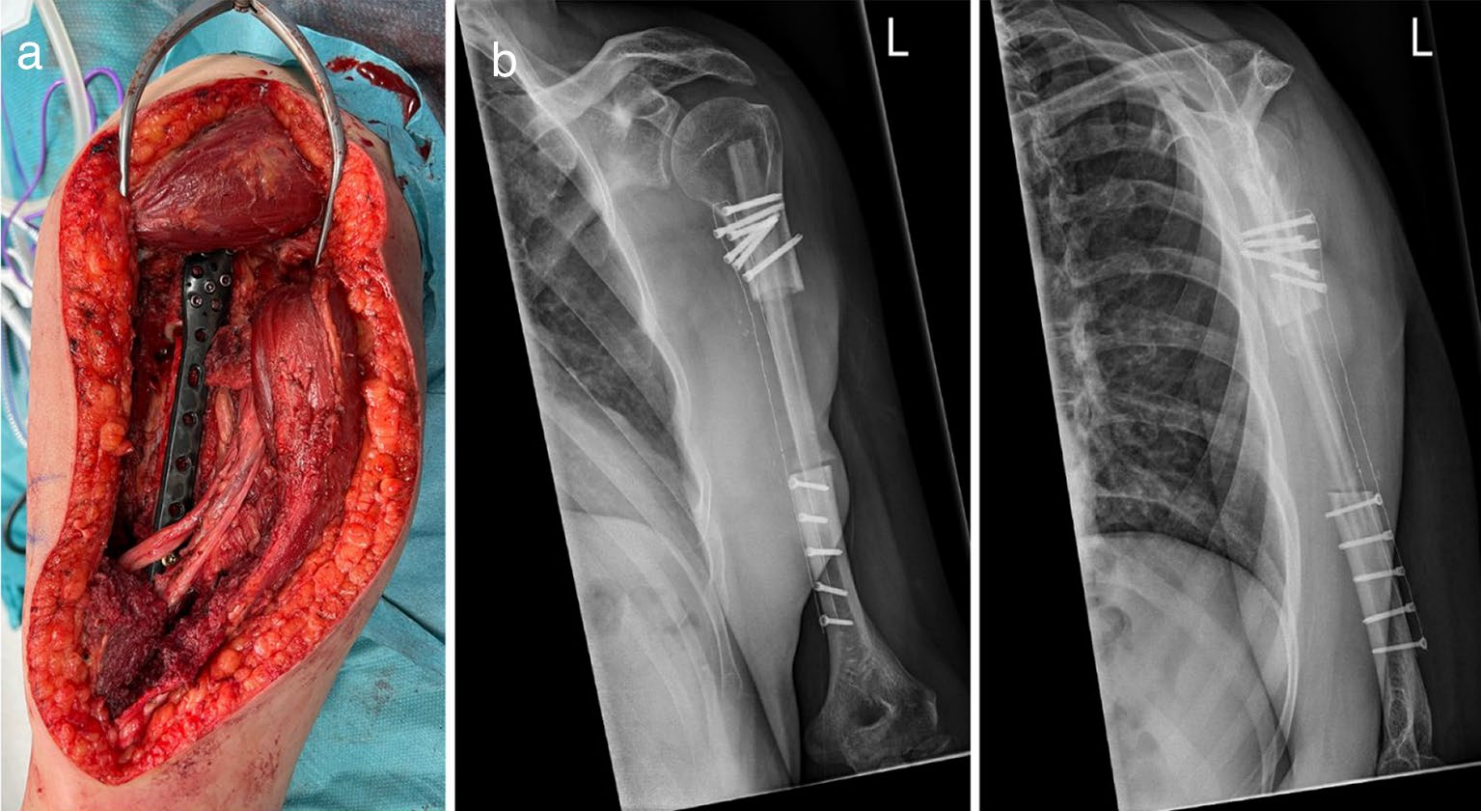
Intraoperative picture after midshaft resection of the left humerus and partial resection of the triceps muscle due to an Ewing Sarcoma. Reconstruction was performed with a free vascularized fibula graft and a carbon-fiber humerus plate (a). Postoperative anteroposterior X-rays of the same patient (b).
